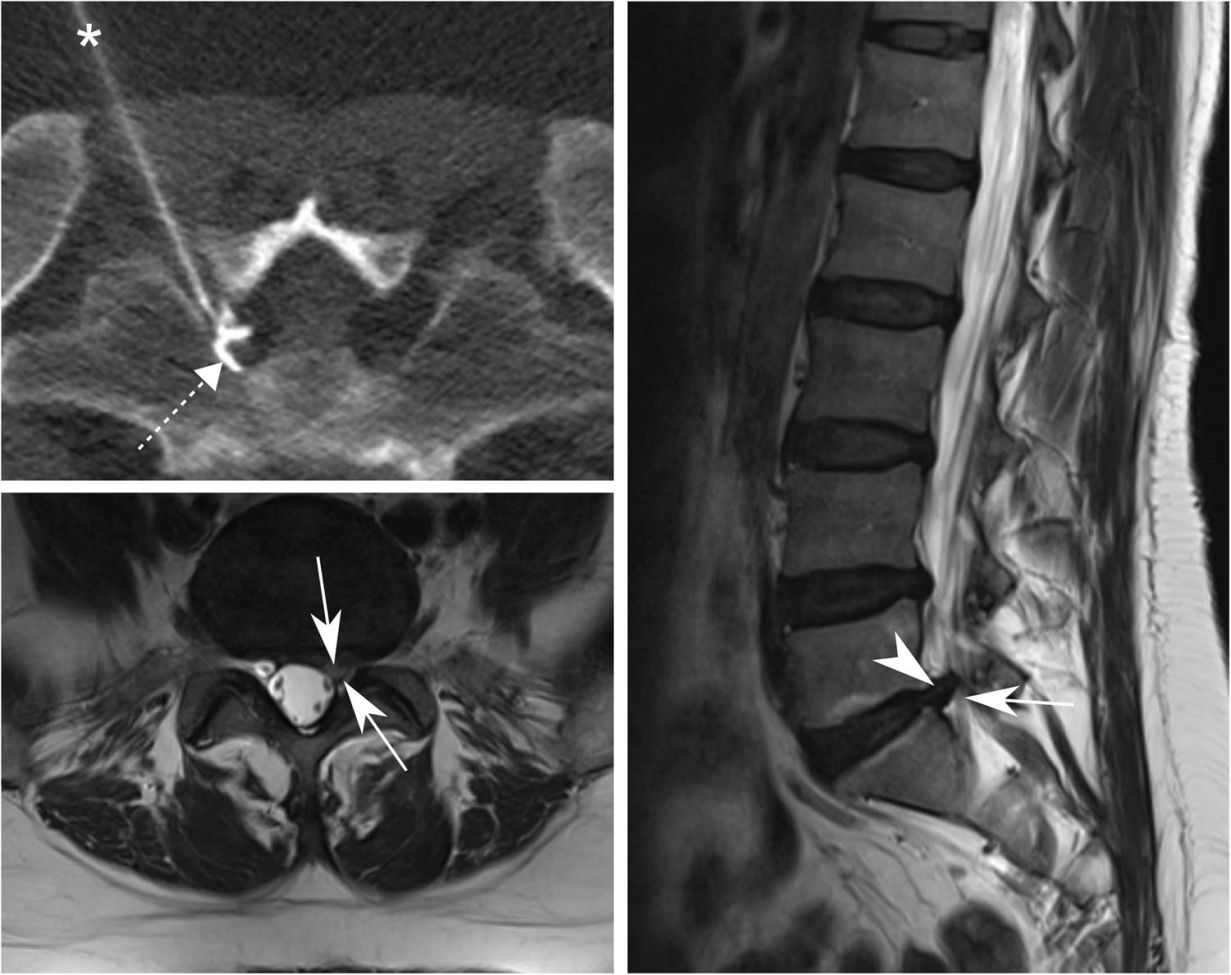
CT-guided TFESI for S1 radiculopathy. A 45-year-old woman with left-sided S1 radiculopathy due to disc herniation (arrowhead) at the L5-S1 level with concomitant grade 2 lateral recess stenosis of the left S1 nerve root (arrows) depicted by transverse (bottom left) and sagittal (right) T2-weighted MR images. CT-guided TFESI (top left) of the S1 nerve root left is shown with correct needle (asterisk) position with the tip adjacent to the nerve root and contrast dispersion (dashed arrow) around the nerve root. *TFESI*, transforaminal epidural steroid injection
